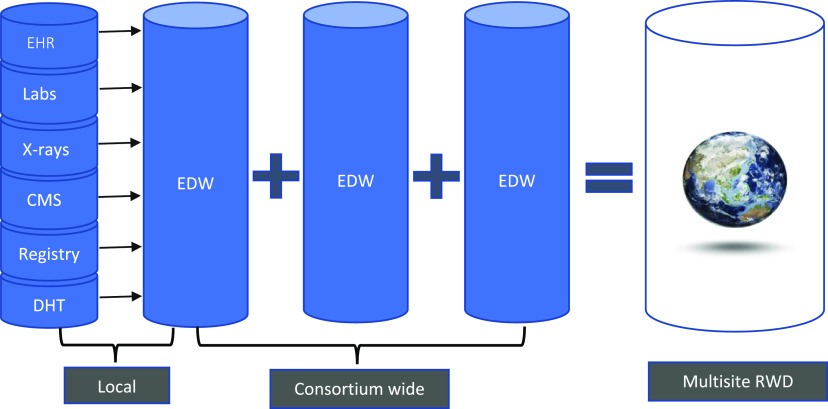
Overall data infrastructure, sources, and integration. CMS = centers for medicare & medicaid services claims data; DHT = digital health technologies; EDW = enterprise data warehouse; HER = electronic health records; Labs = laboratory values; X-ray images; RWD = real-world data.
